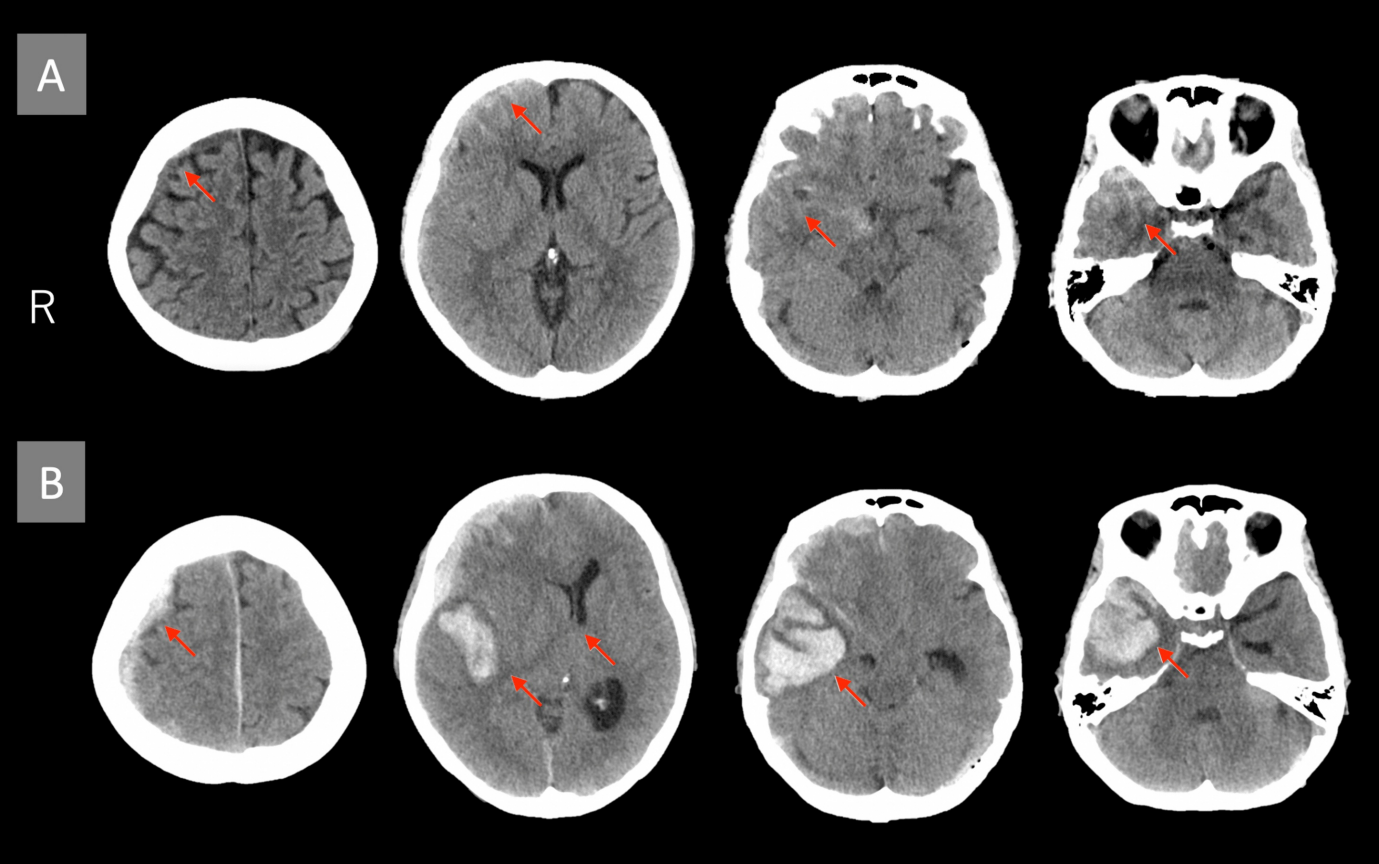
Initial and follow-up head CT scans (A) Initial head CT showing traumatic subarachnoid hemorrhage and acute subdural hematoma (arrows). (B) Follow-up head CT showing a hematoma in the right temporal lobe with midline shift (arrows). CT: computed tomography
